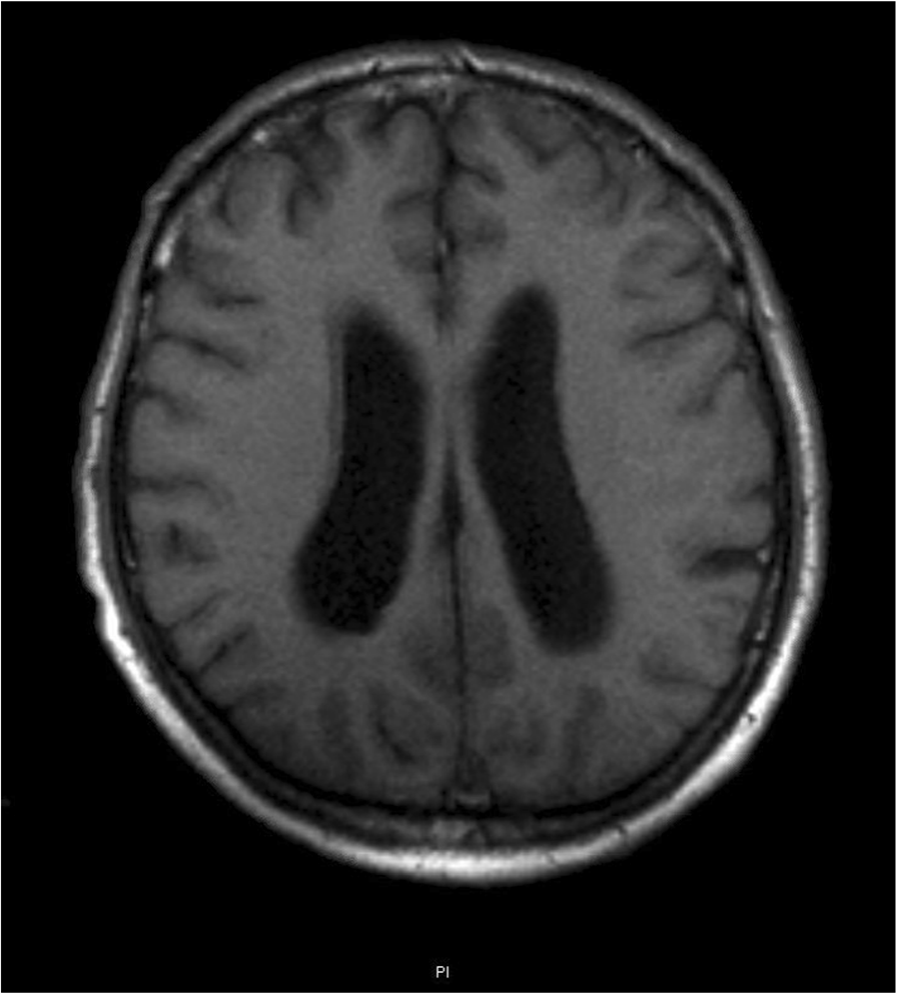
Axial T1 image of MRI Brain showing mild ventriculomegaly
